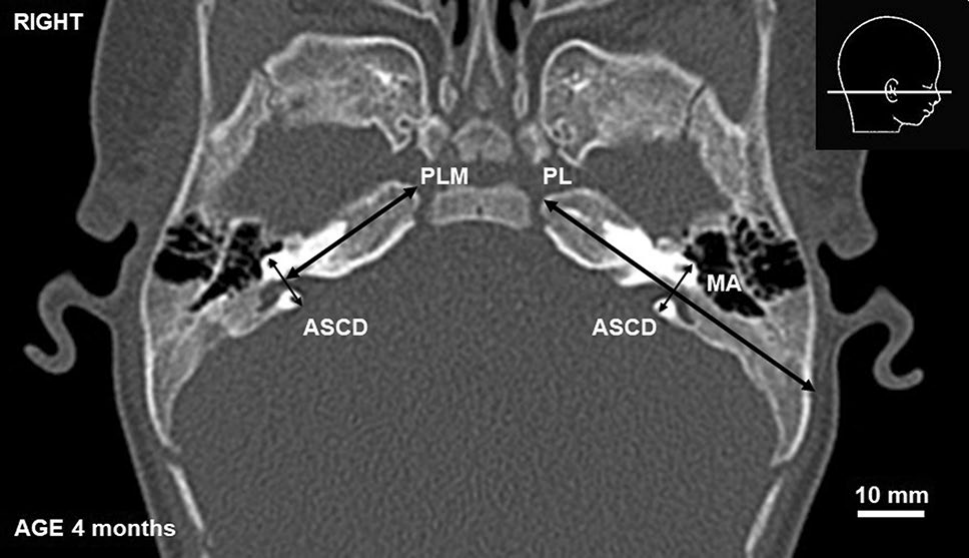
CT scan of a 4-month-old infant. *MA* mastoid antrum, PL, PLM, ASCD—parameters described in the text and Table 1
